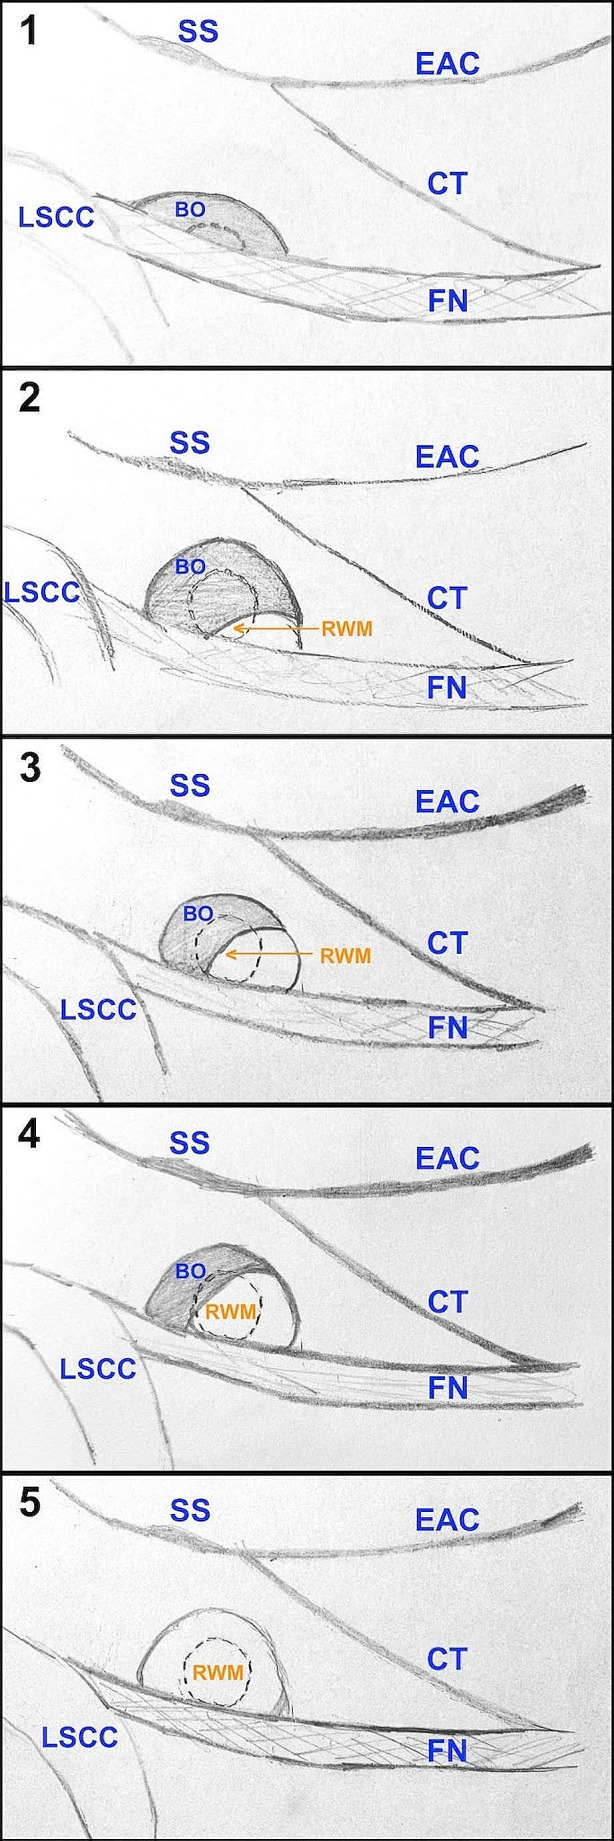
Clinical assessment of RWM visibility. RWM - round window membrane; BO - bone overhang; FN - facial nerve; LSCC - lateral semicircular canal; CT - chorda tympani; EAC - external auditory canal; SS - suprameatal spine
